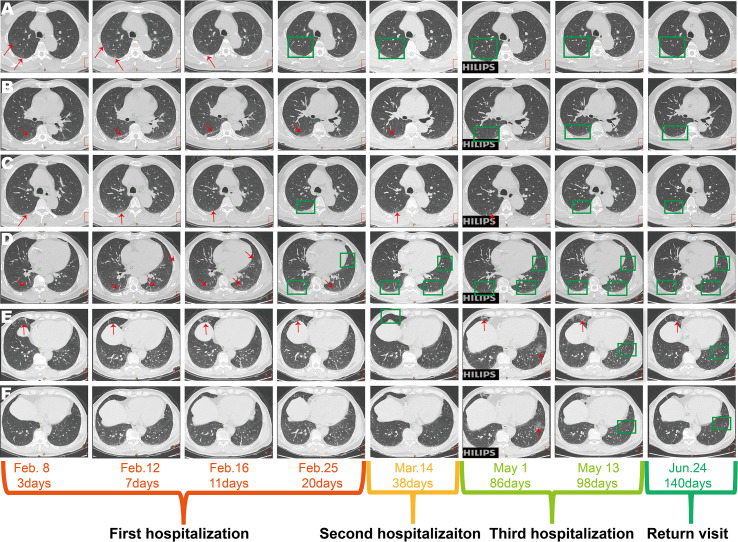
Chest computed tomography scans of the COVID-19 patient with SARS-CoV-2 RNA re-tested positivity during the hospitalization period. (A) The ground-glass opacities in the upper part of the posterior segments of the right upper lobe. (B) The ground-glass opacity in the posterior segments of the right lower lobe. (C) The ground-glass opacity in the lower part of posterior segments of the right upper lobe disappeared in the first hospitalization, re-appeared in the second and third hospitalization (the first recurrent SARS-CoV-2 RNA positive and the second recurrent SARS-CoV-2 RNA positive). (D) The ground-glass opacity in the right upper lobe, left lower lobe, and lingual segment of the left upper lobe. (E) The lesion of the right middle lung displayed as patchy, ground-glass and linear lesion during the first hospitalization, disappeared during the second hospitalization, re-appeared and was enlarged during the third hospitalization, but was absorbed on the return visit (June, 24, 2020). (F) Appearance of the ground-glass opacity in the basal segment of the left lower lung near the diaphragmatic dome at third hospitalization (the second recurrent SARS-CoV-2 RNA positive).
